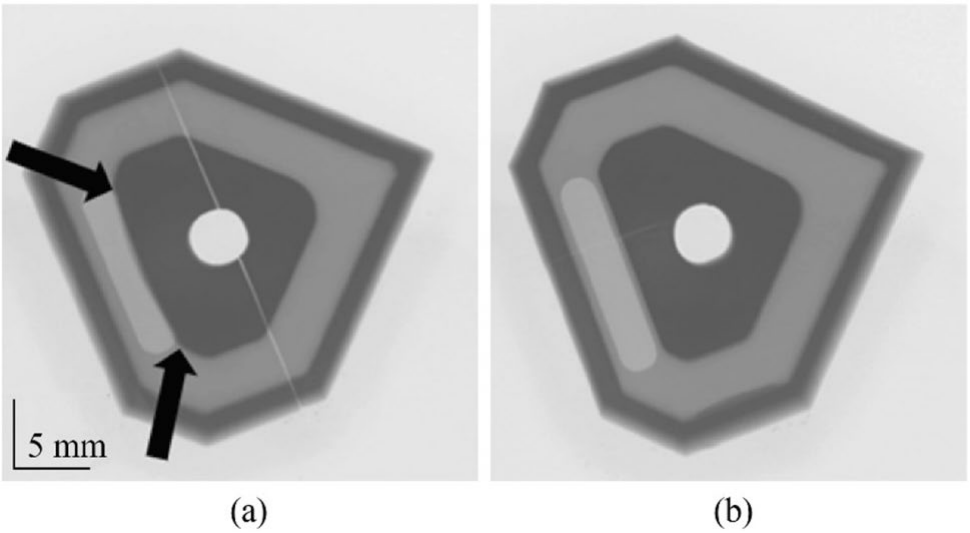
Micro X-ray images showing a a linear fracture present in sample and b not observed in sample (the arrows highlight the bulging effect reflecting the different orientation used in the LCM process)
